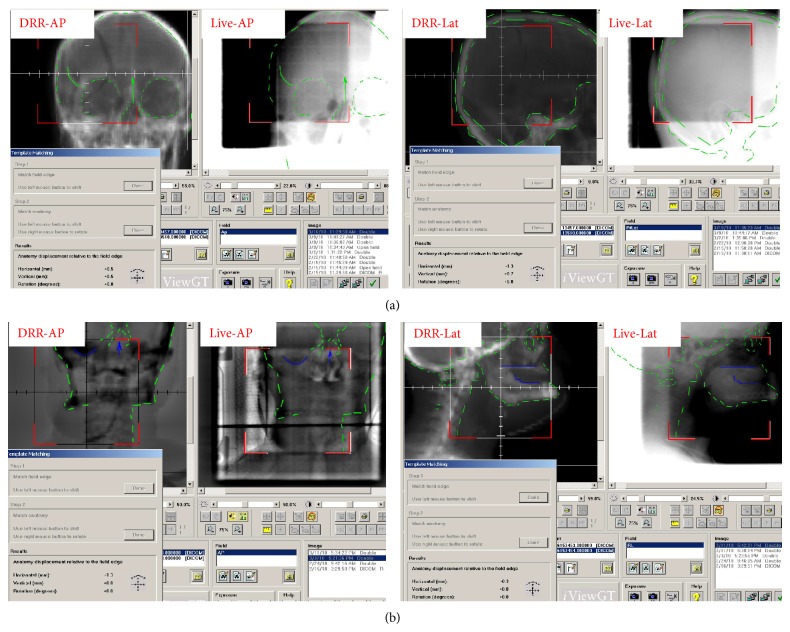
Use of MV EPID for online correction using orthogonal 2D images (anteroposterior and lateral). Both the field and the bony anatomy are matched sequentially to give an estimate of error. The comparison of live image with reference DRR helps assess and correct translational shifts but does not estimate rotational errors. (a) Right parietal glioma. (b) Head and neck cancer. MV: Megavoltage; EPID: Electronic portal imaging device; 2D: two-dimensional; DRR: Digital reconstructed radiograph.
